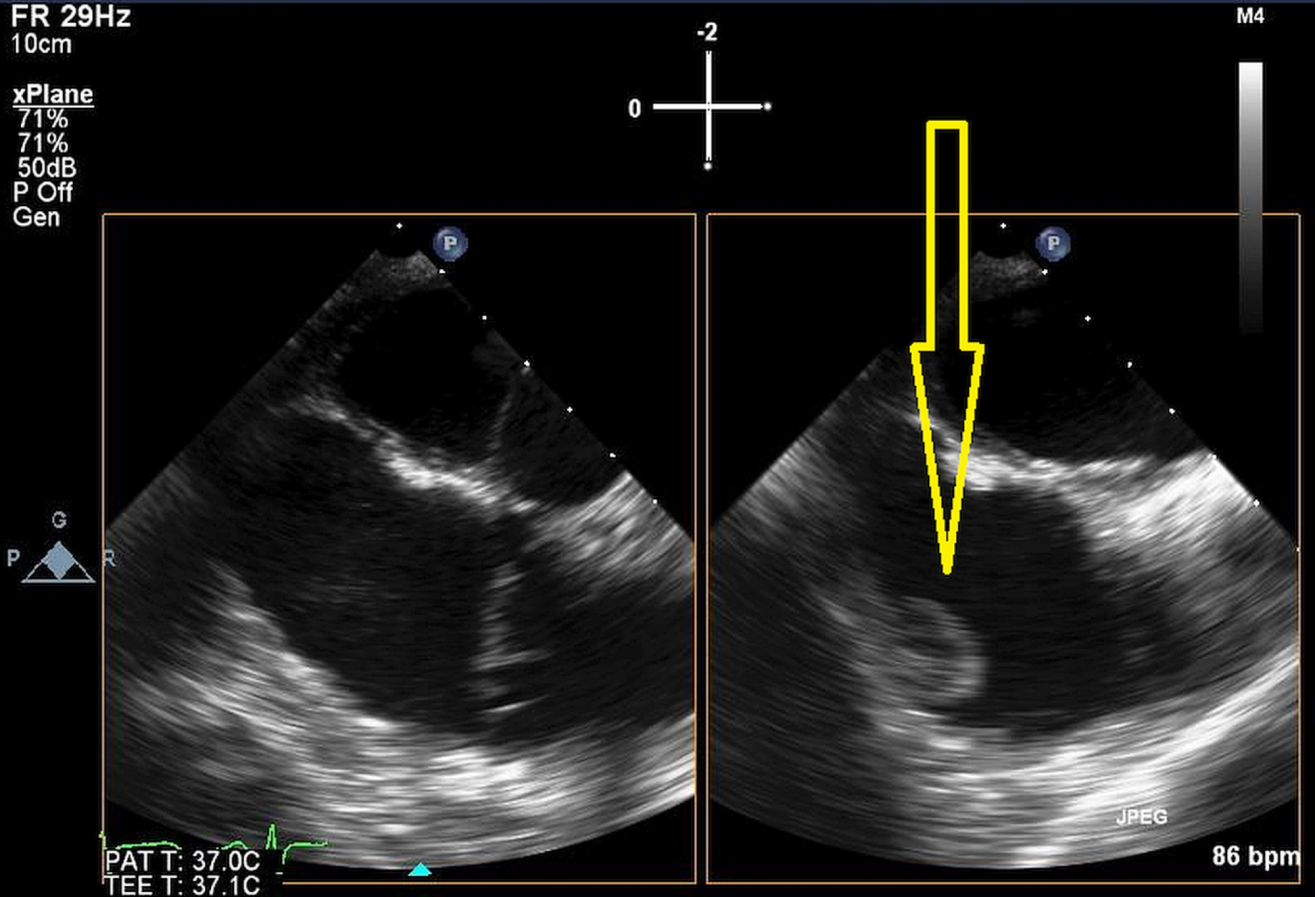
Transthoracic echocardiogram (TTE) revealed a right atrial mass measuring 1.5 cm x 2 cm. Arrow as shown displays position of right heart mass.
